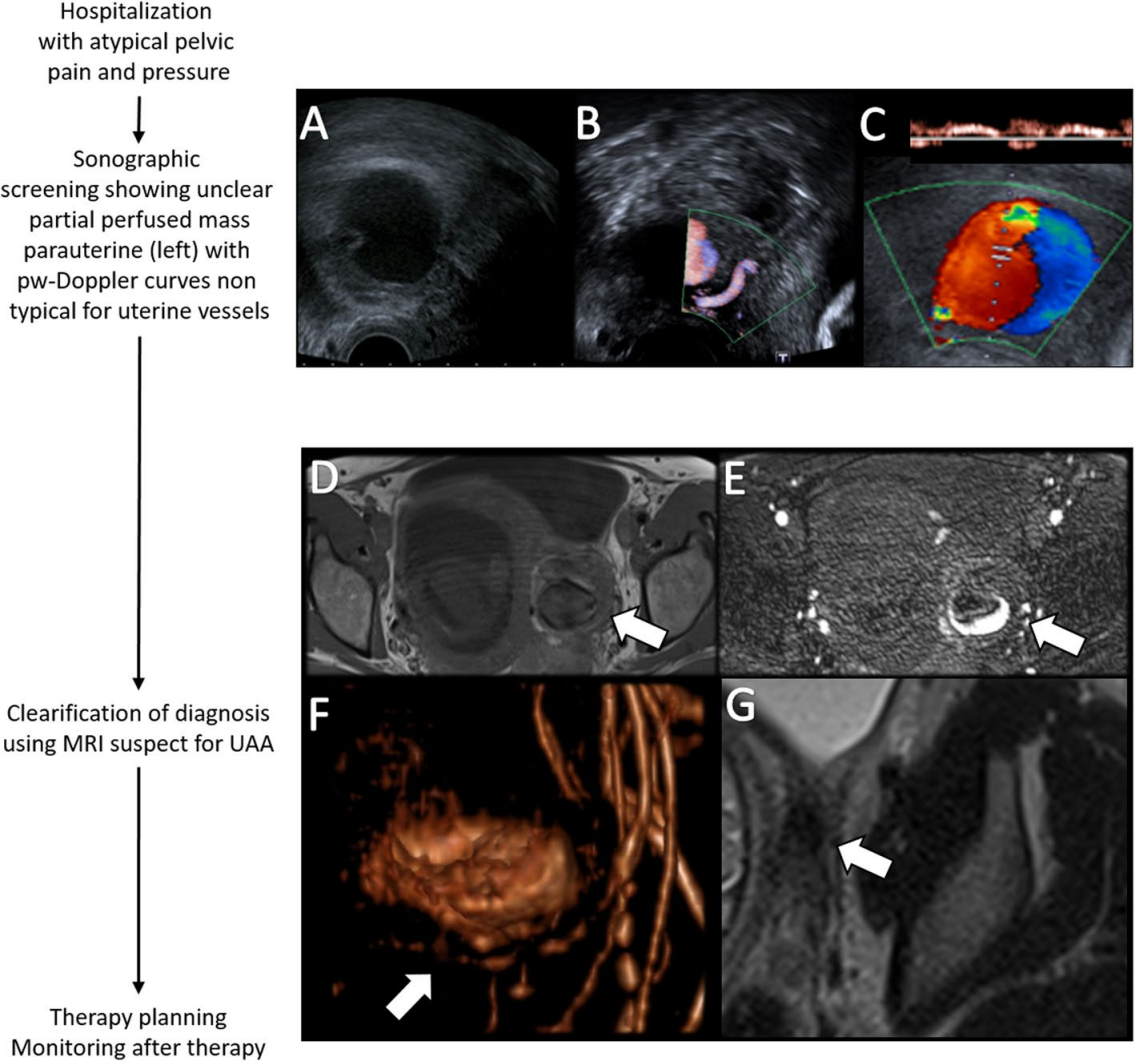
Pregnant women presented with untypical pelvic pain and pressure showing a hypoechoic parauterine mass at B-Mode sonography measuring 39 mm (A). Positional relation to the uterine artery (B) and intralesional flow at color-coded sonography with pw-Doppler curves non typical for uterine vessels and typical yin-yang sign for an aneurysmatic lesion (C). Clarification of diagnosis suspect for UAA using contrast enhanced MRI (D-F). Contrast enhanced QUISS-sequence showing intra-aneurysmatic collection of contrast agent (E, arrow) comparing to native T1w-sequence (D, arrow). MR angiography shows the exact anatomy of the internal iliac artery with the true aneurysm originating from the uterine artery (F, arrow). Control MRI before the calculated date of birth showed a significant decreased and still occluded aneurysm (G, arrow)
